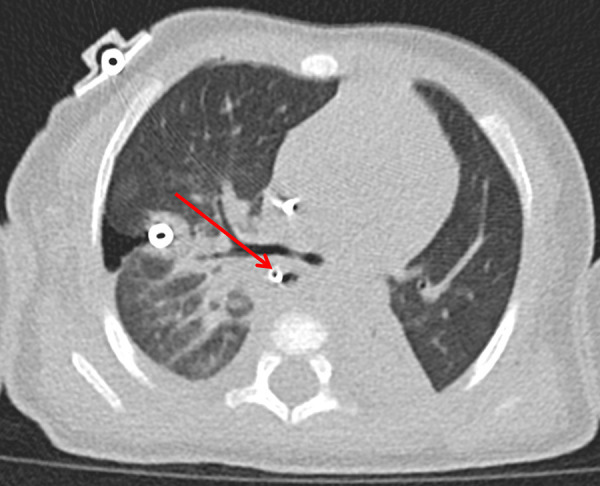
High-resolution CT. 1. A tube is visible in the right chest wall and pleural cavity; small right-sided pneumothorax with approximately 20% compression of the right lung. 2. A tubular shadow is visible within the oesophagus, an air-filled balloon-like shadow protruding from the right side of the middle segment of the oesophagus outside the oesophageal contour. 3. Bilateral pneumonia; partial consolidation and atelectasis in the lower lobe of the left lung.
